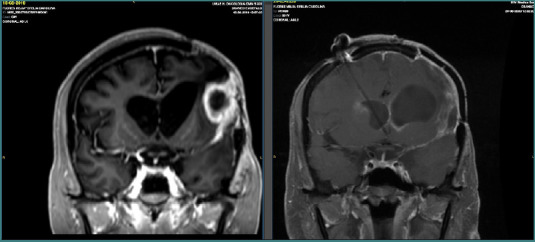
Coronal MRI images before and after multimodal treatment
